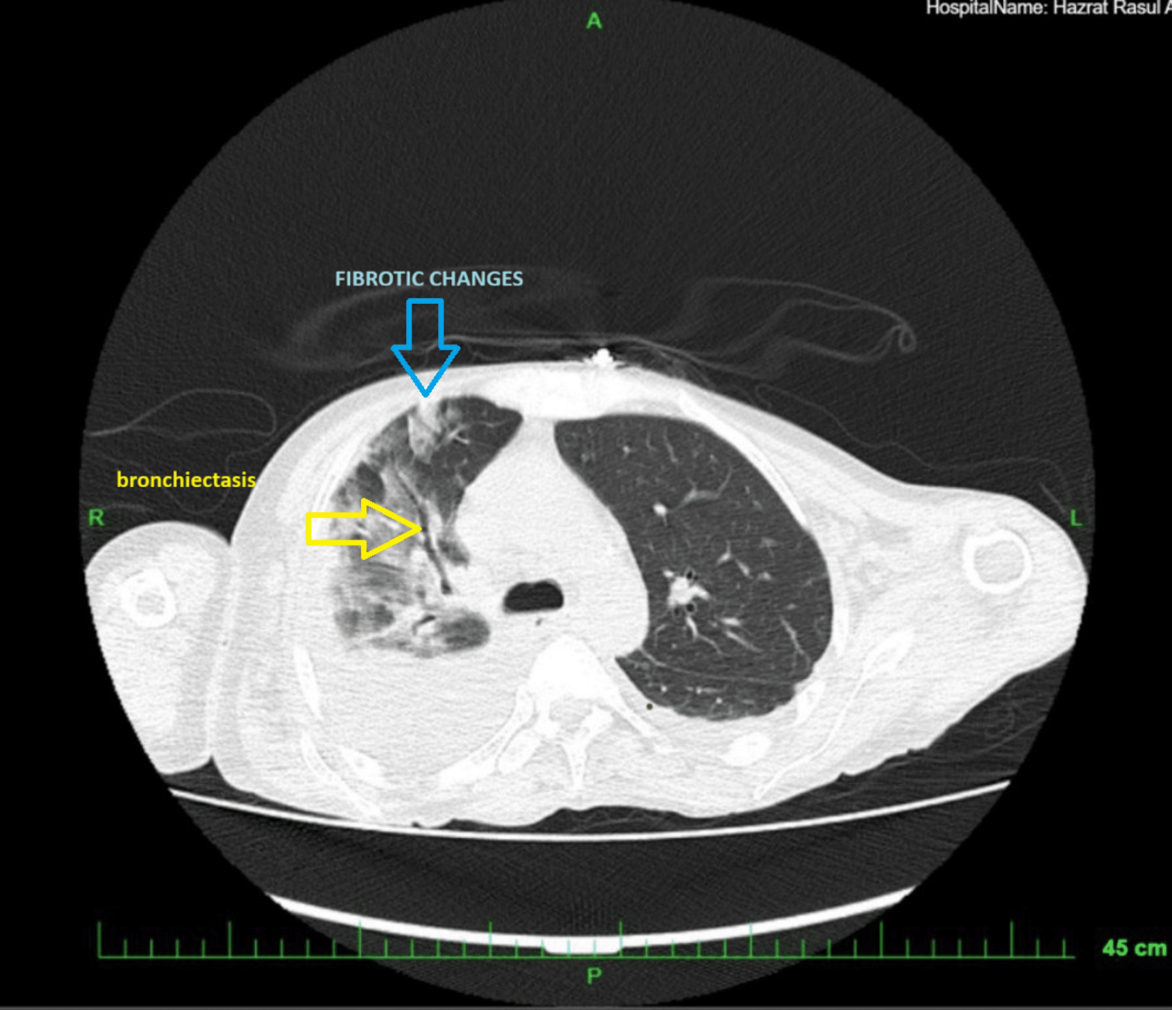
Computed Tomography (CT) scan showing bronchiectasis and fibrotic changes in the right lung.
